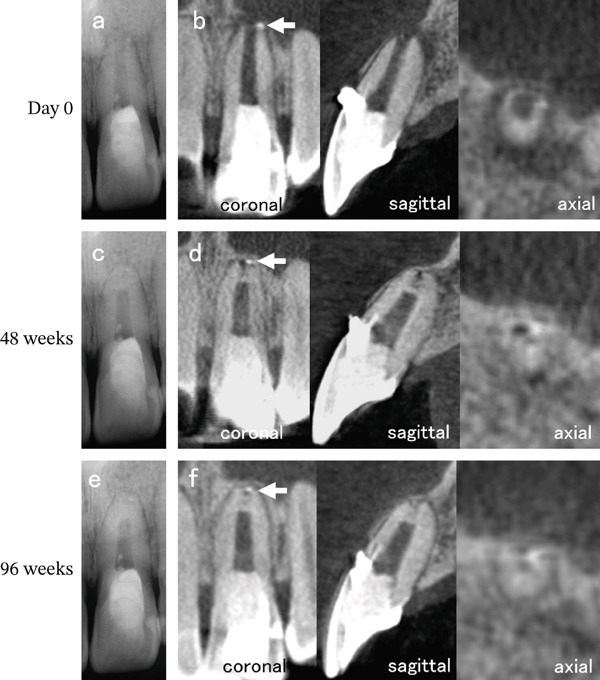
Case 1. Time‐course radiographic and CBCT evaluation of the maxillary left central incisor following DPSC transplantation. (a, c, e) Periapical radiographs and (b, d, f) CBCT images in coronal, sagittal, and axial views obtained immediately after transplantation (Day 0; a, b) and at (c, d) 48 and (e, f) 96 weeks postoperatively. Arrows indicate the periapical region containing the previously extruded radiopaque material. Day 0: Baseline status immediately after transplantation; periapical radiolucency and the radiopaque material extruded beyond the apex are visible. 48 weeks: resolution of the periapical radiolucency, apical mineralization within the root canal, and apparent inward displacement of the extruded radiopaque material into the canal space. 96 weeks: Further progression of apical mineralization with sustained absence of periapical radiolucency. Clinically relevant findings: progressive healing of apical periodontitis and intracanal mineralized tissue formation, consistent with favorable healing following the regenerative procedure.
